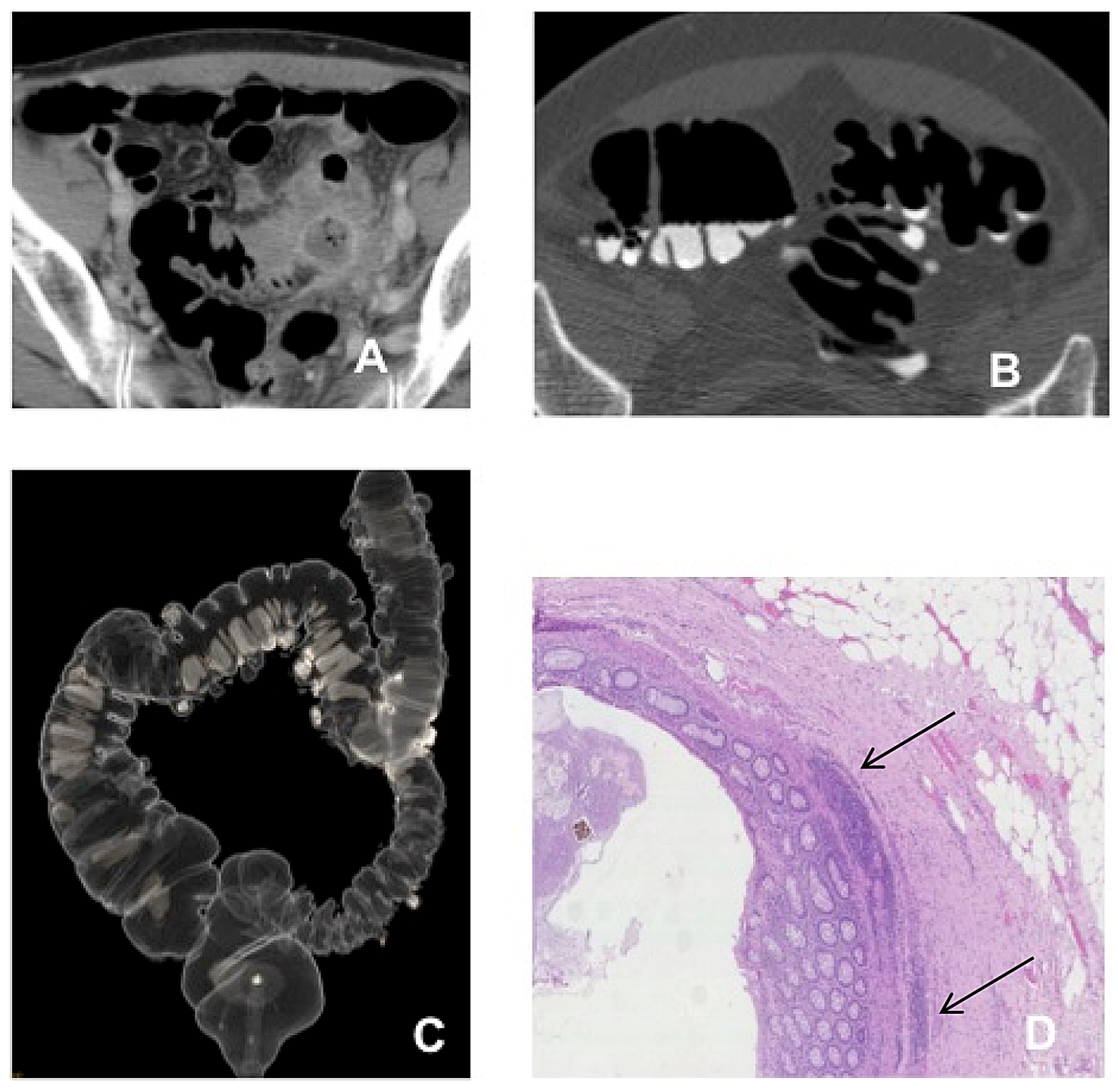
Images in a 59-year-old-man who underwent elective surgery after recovery from acute diverticulitis. Axial two-dimensional (2D) supine conventional computed tomography (CT) a shows acute diverticulitis in the presence of sigmoid colon wall thickening, mesenteric fascial thickening and small abscess (complicated AD). Axial 2D supine computed tomography colonography (CTC) image b and DCBE CTC like-view c images showing sigmoid colon diverticula without wall thickening or significant lumen stenosis (diverticular disease severity score [DDSS] 1). In this whole-thickness section of the colonic wall (d), only scarce fibrosis and focal chronic inflammation (arrows) is appreciable
